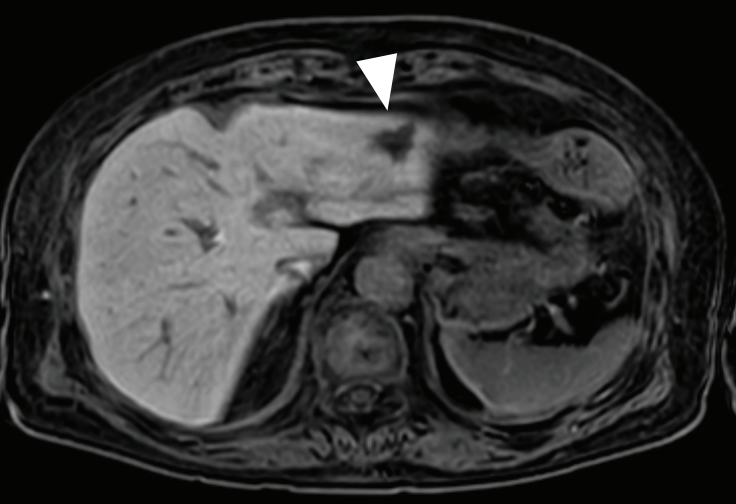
Gadoxetic acid–enhanced MRI performed before hepatectomy. The image demonstrates the identified liver lesion in the lateral segment, showing reduced gadoxetic acid uptake (arrowhead), without evidence of additional hepatic metastases.
